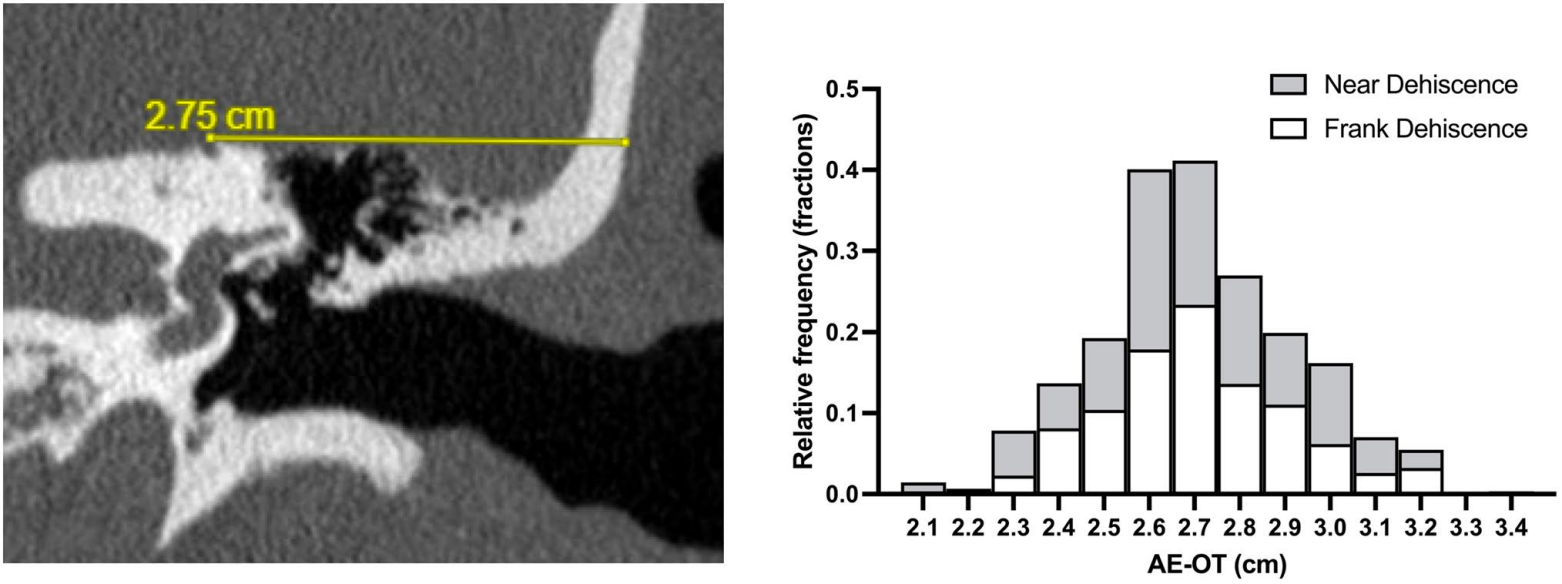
Measurement of the distance between arcuate eminence and temporal bone outer table on computed tomography imaging (left) and distribution histogram of distance between arcuate eminence and outer table of the temporal bone (right)
